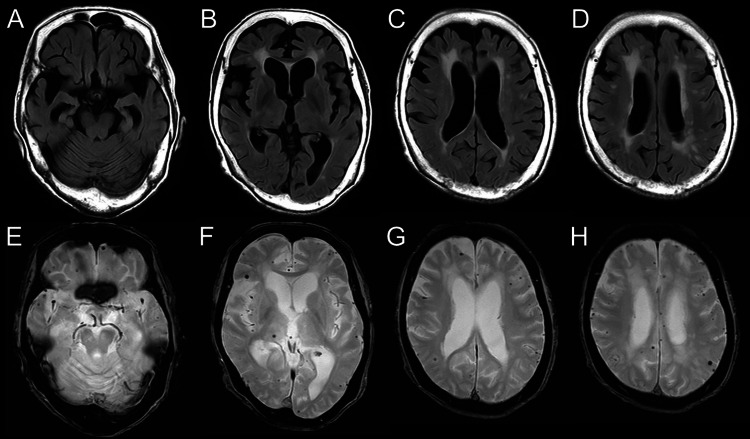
Follow-up head MRI at 6 months (A–D) Fluid-attenuated inversion recovery (FLAIR); (E–H) T2*-weighted imaging FLAIR hyperintense lesions previously observed in the cerebellum and bilateral cerebral white matter had resolved. However, multiple cerebral microbleeds persisted in the right thalamus and bilateral cortical and subcortical regions.
